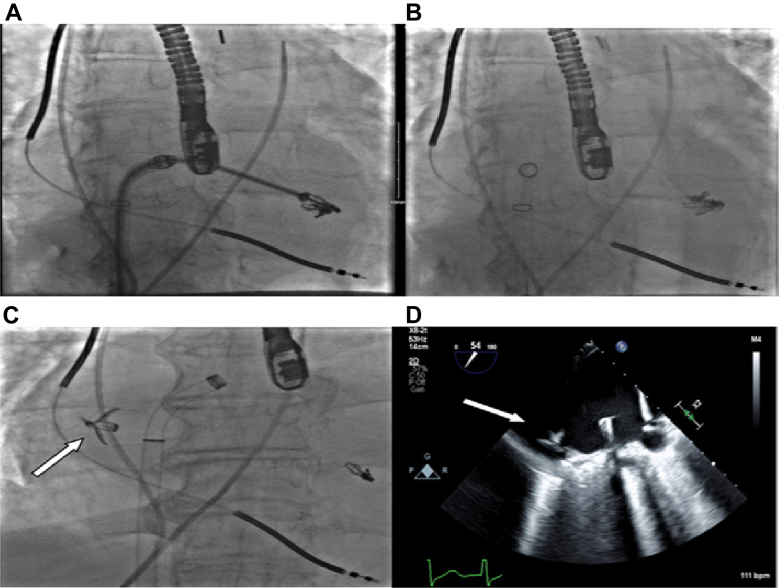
Fluoroscopic and Echocardiographic Images Depicting Events Leading to Embolization of MitraClip Into Right Lower Pulmonary Vein Fluoroscopic images showing (A) second MitraClip deployment next to the first MitraClip, with the delivery system across the mitral valve; (B) the delivery system retracted, with the second clip unstable next to the first clip on fluoroscopy; and (C) the second MitraClip embolized into the right lower pulmonary vein (arrow). (D) Two-dimensional echocardiogram showing the embolized MitraClip in the lower right pulmonary vein.
